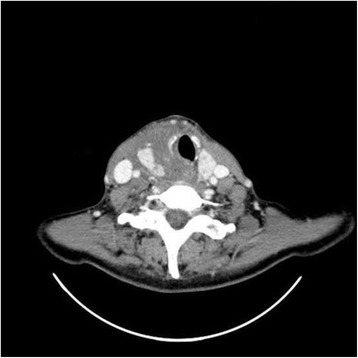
Contrast-enhanced computed tomography revealed a laceration of the right thyroid lobe with diffuse hematoma
